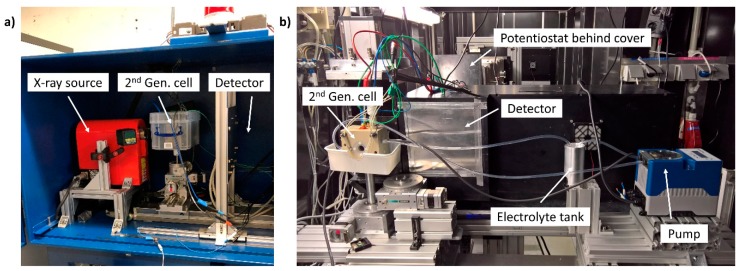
Photographs of the experimental in-operando set-up (a) micro-computed tomography at Helmholtz-Zentrum Berlin; (b) neutron radiography at CONRAD2 Berlin.
